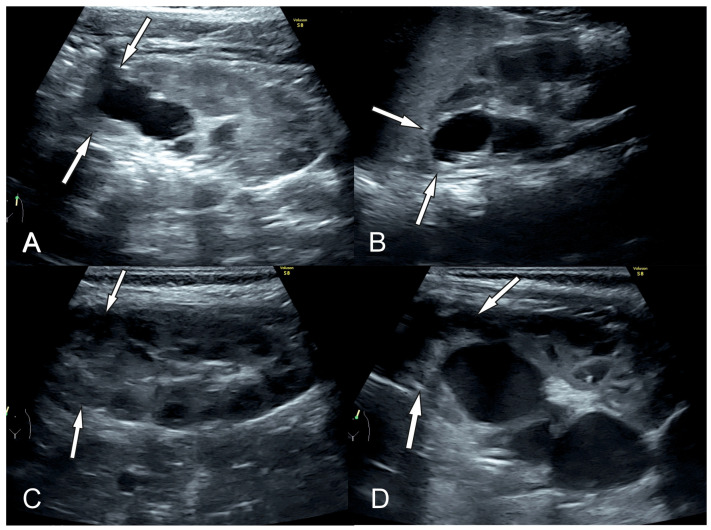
An example of ultrasound findings in two patients ((A,B) patient Nr 5R,5L; (C,D) patient Nr 12) with bilateral duplex kidneys with suspected segmental dysplasia. (A,B) Reduced parenchymal thickness and increased parenchymal echogenicity bilaterally (arrows). On the right, CMD of the upper pole is reduced (A) and absent in the left kidney (B). (C) The parenchymal thickness and CMD of the right kidney are preserved, inhomogeneous increased echogenicity of the upper pole. The right kidney was not operated. (D) The image presents reduced parenchymal thickness and increased parenchymal echogenicity of the upper pole in the left kidney; CMD is reduced.
